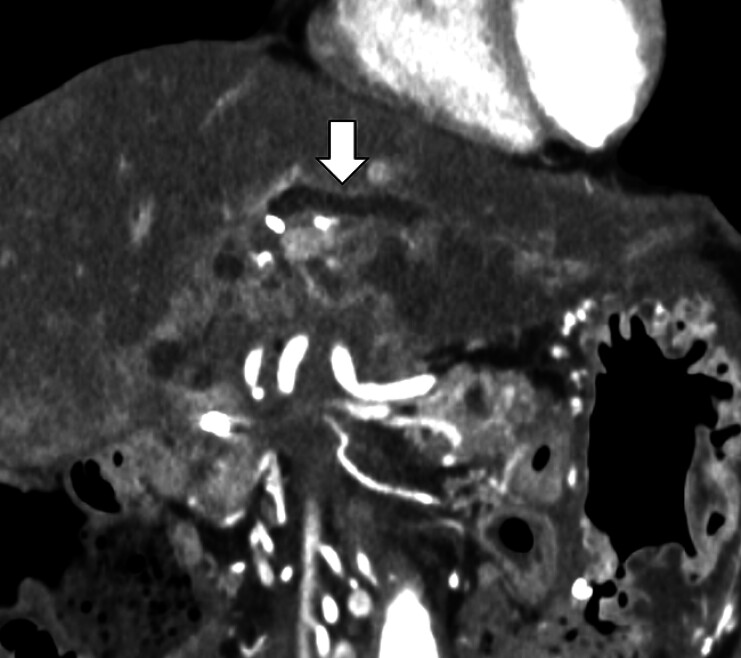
Computed tomography revealed dilated bile ducts (arrow), consistent with cholangitis after pancreaticoduodenectomy.
